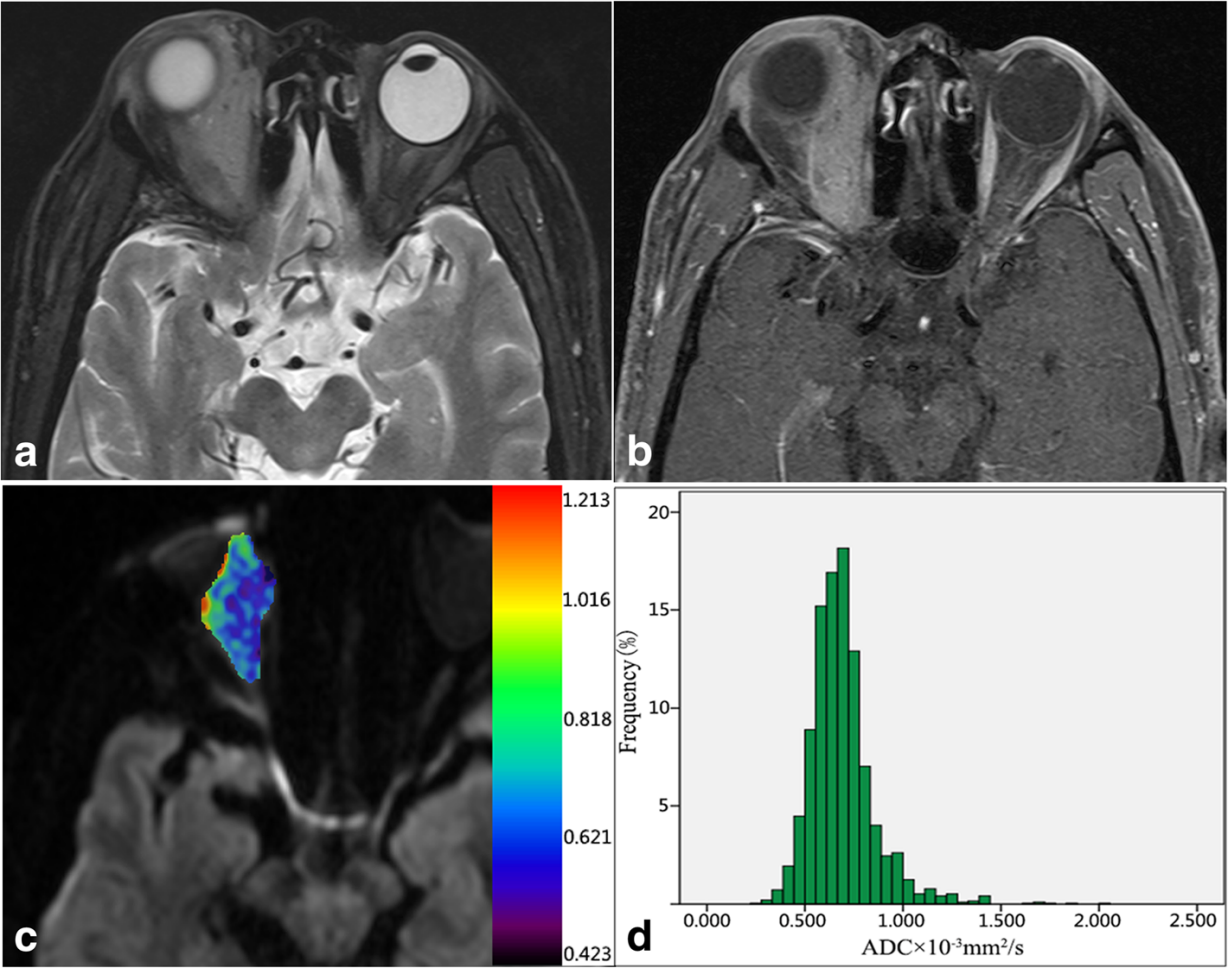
A male patient with MALT lymphoma. Axial fat-saturated T2W image a shows a well-defined isointense mass involving intra and extraconal space and extending to the orbit preseptal space. Axial contrast enhanced T1W image b demonstrates homogenous moderate enhancement, lower than that of extraocular muscles. Pixel-by-pixel colored ADC map c was obtained and then embedded with the axial DWI. The corresponding ADC histogram from VOI d shows a top peak and a slight tail on the right. Whole-tumor mean ADC was 0.692 × 10^− 3^ mm^2^/s, ADC_10_ was 0.520 × 10^− 3^ mm^2^/s, skewness was 1.81, and kurtosis was 7.51
